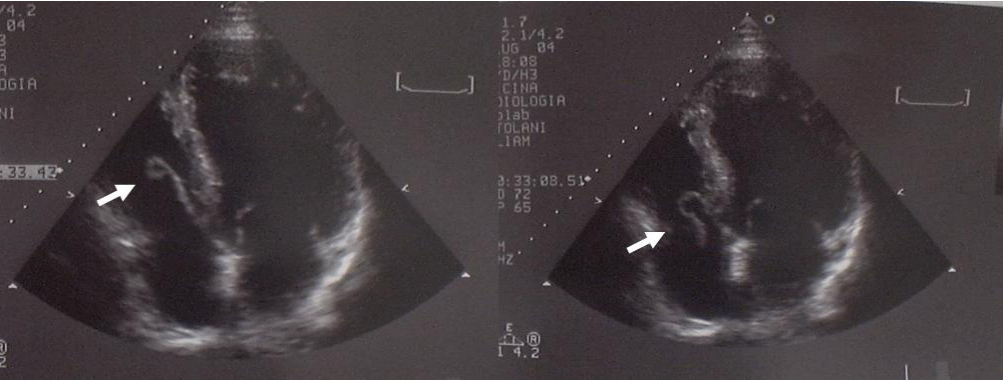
Transthoracic echocardiogram showing a vegetation on the septal leaflet of the tricuspid valve.
